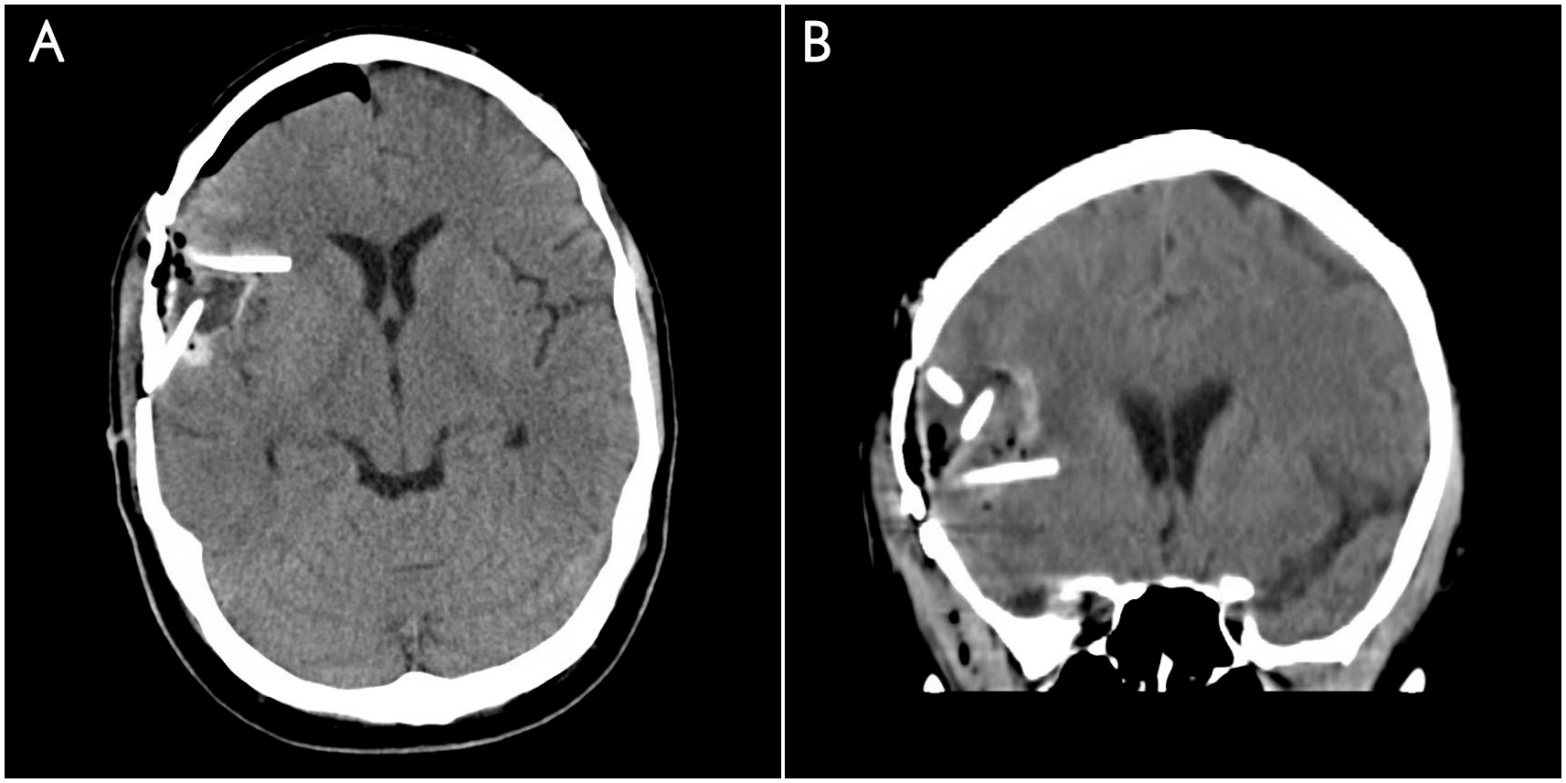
Postoperative imaging. Representative (A) axial and (B) coronal planes of a CT head on postoperative day 0 demonstrating postsurgical changes and three catheters traversing the lesion and entering the surrounding parenchyma.
